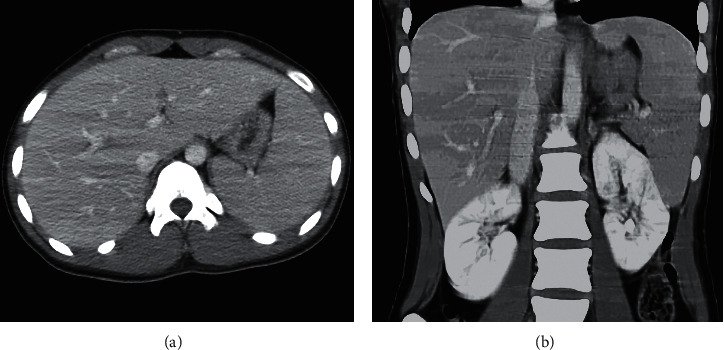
Abdominal contrast-enhanced CT upon admission. (a) Axial view; (b) Coronal view. There is splenohepatomegaly.
